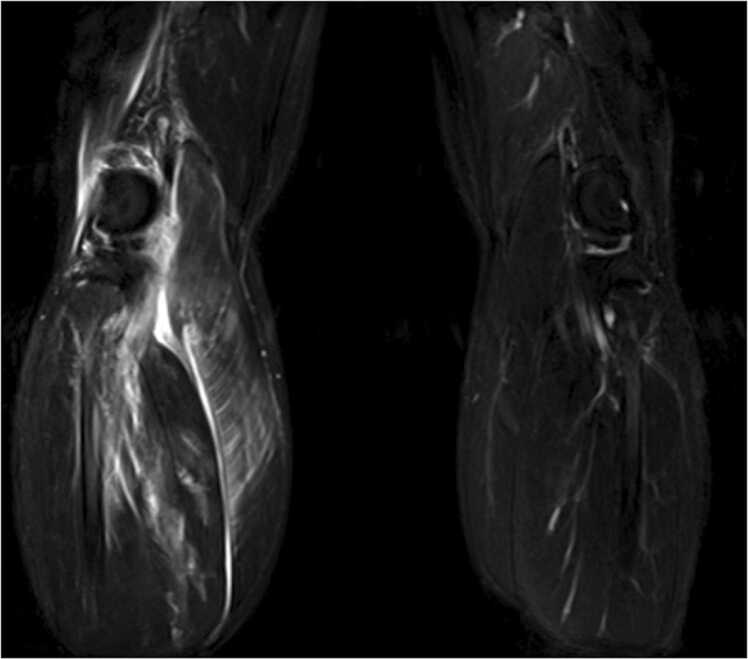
T2-weighted magnetic resonance imaging (MRI). High intensity image shows the fascia in the right popliteal region and intermuscular fluid collection.
